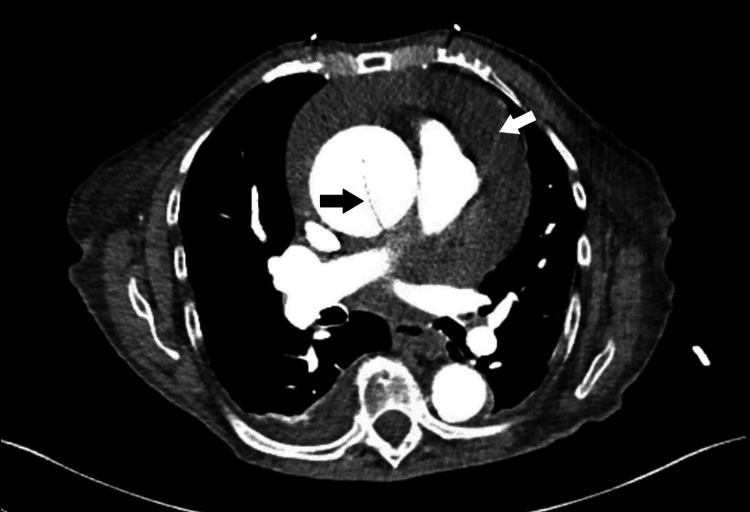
CTA of the chest demonstrating aneurysmal dilatation of the ascending thoracic aorta with a dissection flap extending to the proximal aortic arch (black arrow) as well as a large amount of hyperdense fluid within the pericardium suggestive of hemopericardium (white arrow). CTA: computed tomography angiography
